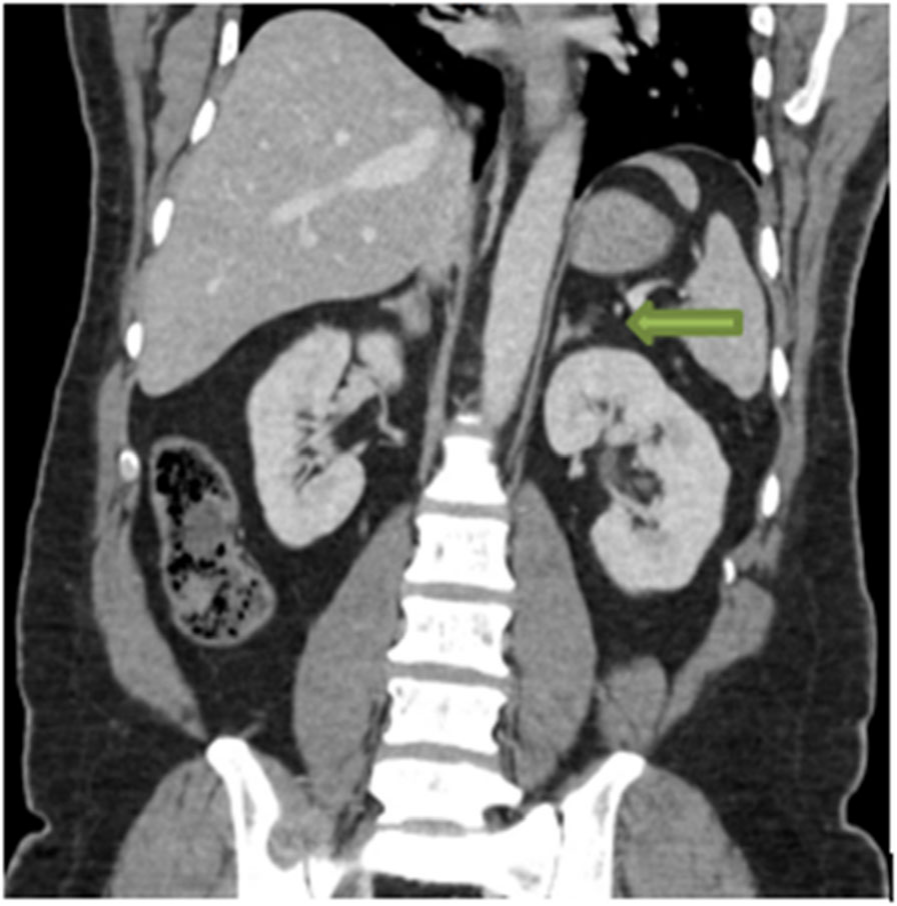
Computed tomography scan of the abdomen showing left adrenal adenoma by the *green arrow*
